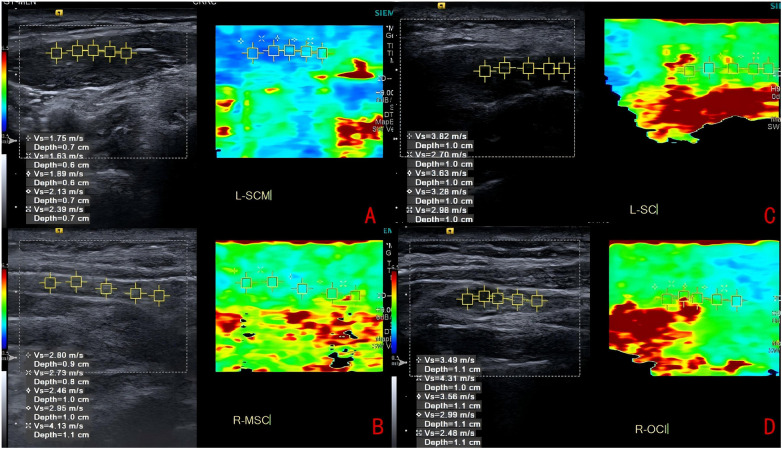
Patient's SWE examination. The figure is ultrasound elastography images of the neck muscles in a patient with cervical vertigo. Labels (A–D) denote the sternocleidomastoid, musculus splenius capitis, semispinalis capitis, and obliquus capitis inferior muscles, respectively. The grayscale images on the left demonstrate anatomical localization, while the corresponding images on the right display tissue elasticity characteristics. In the elastograms, red represents areas of high elastic modulus, and blue indicates areas of low elastic modulus. The continuous color transition from red to blue reflects a corresponding decrease in muscle fiber stiffness.
